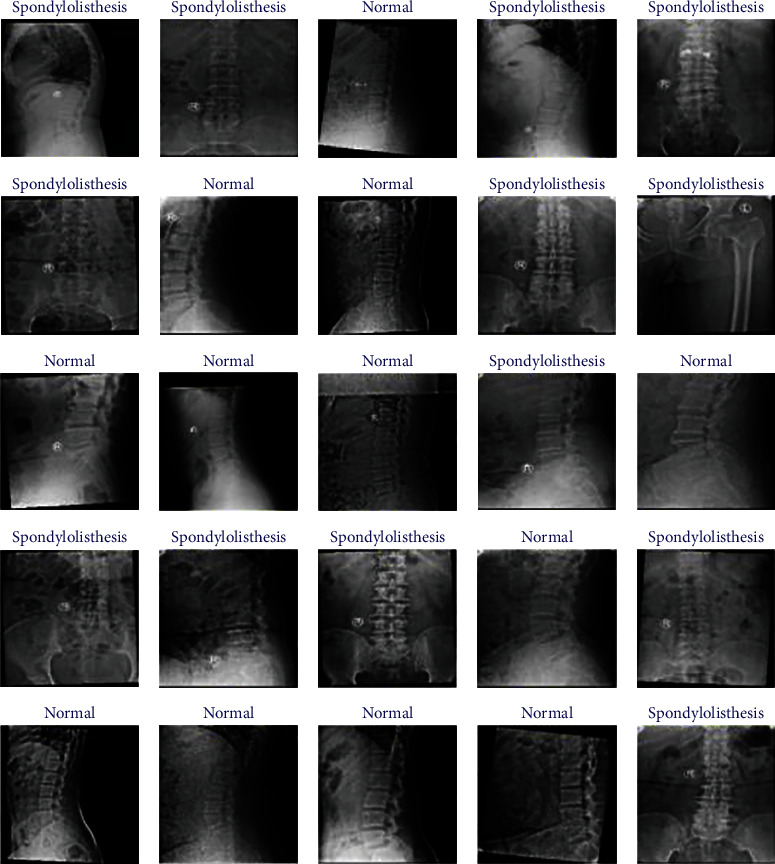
Glimpse of X-ray images from our private dataset.
